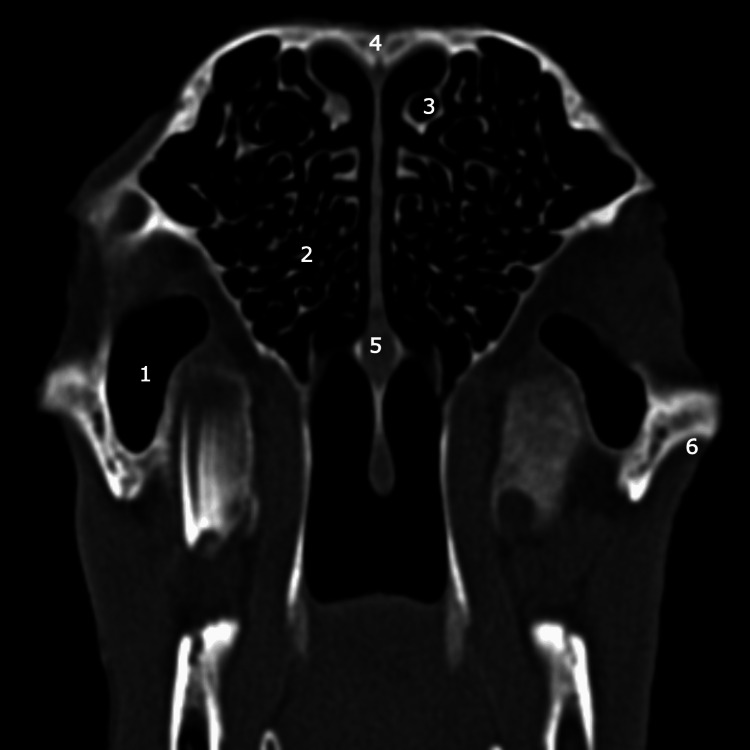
Coronal CT scan: level of the ethmoidal labyrinth 1: maxillar sinus; 2: ethmoidal labyrinth; 3: posterior part of superior turbinate; 4: frontal bone; 5: nasal septum; 6: zigomatich bone.
